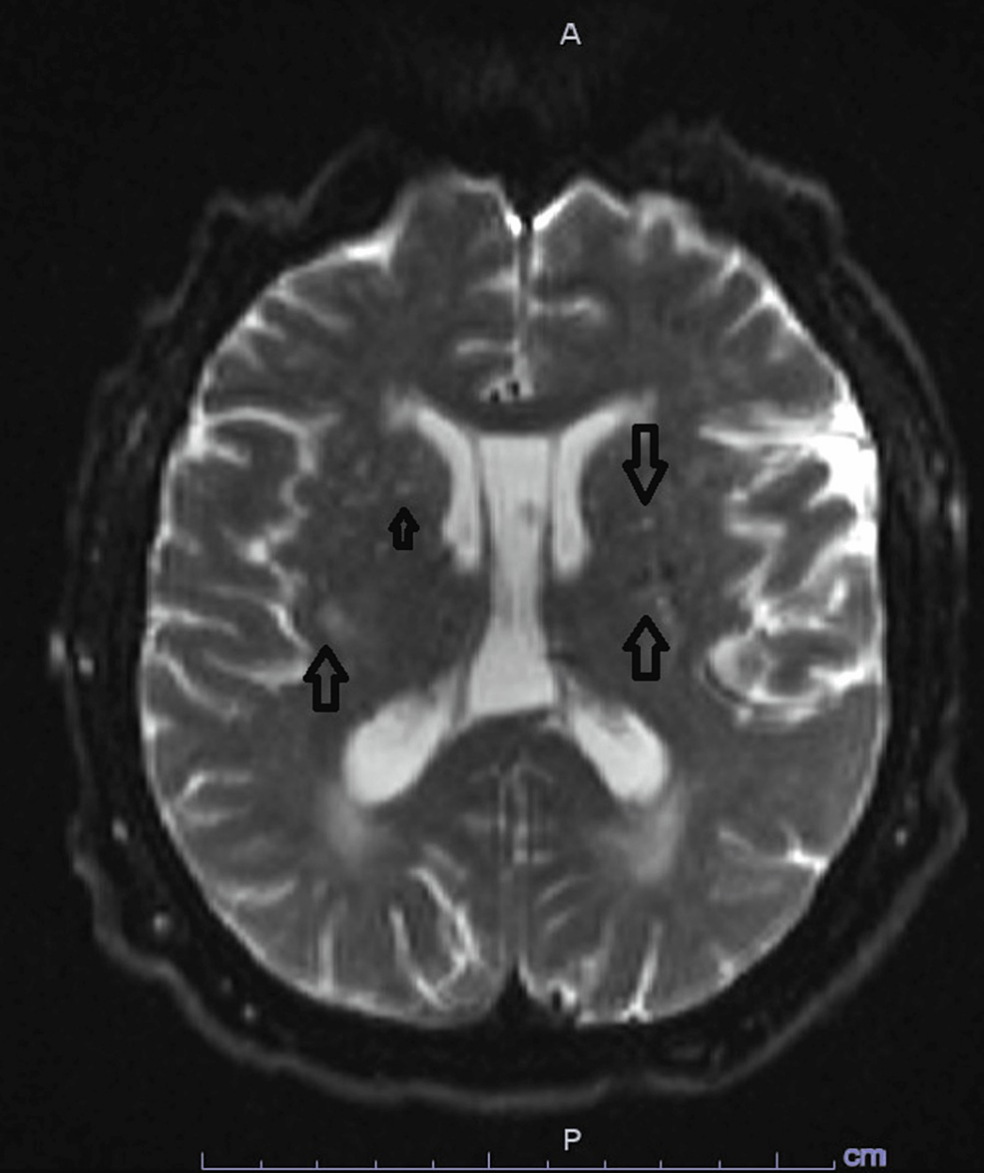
Initial MRI brain with cerebral strokes Magnetic resonance imaging (MRI) brain with and without contrast further delineating the extent of bilateral cerebral hemisphere acute/sub-acute infarctions mainly affecting watershed areas, centrum semiovale, and corona radiata (arrows)
